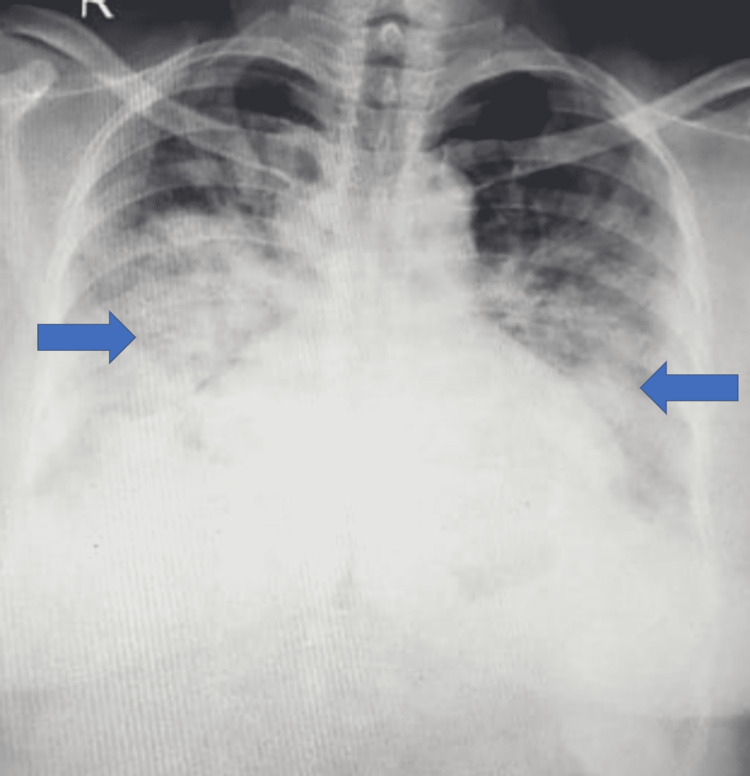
Chest radiograph showing bilateral mid and lower zone opacities (arrows)
